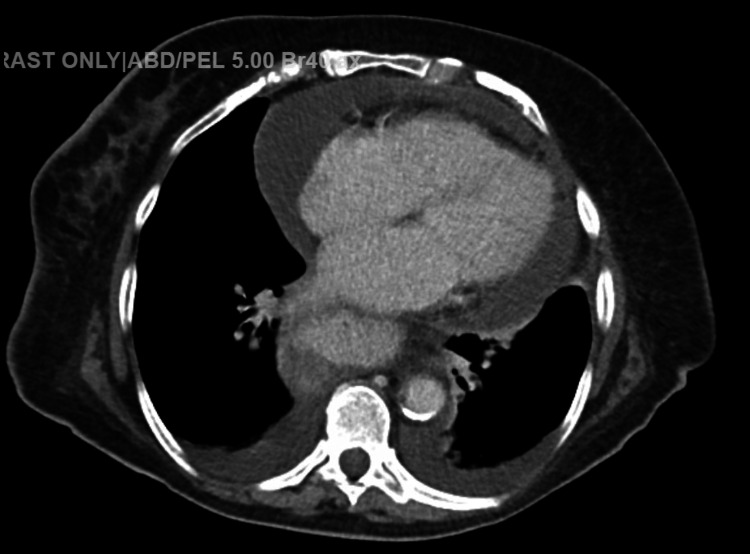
Initial CT angiogram, suggestive of pleural and pericardial effusion CT: computed tomography
